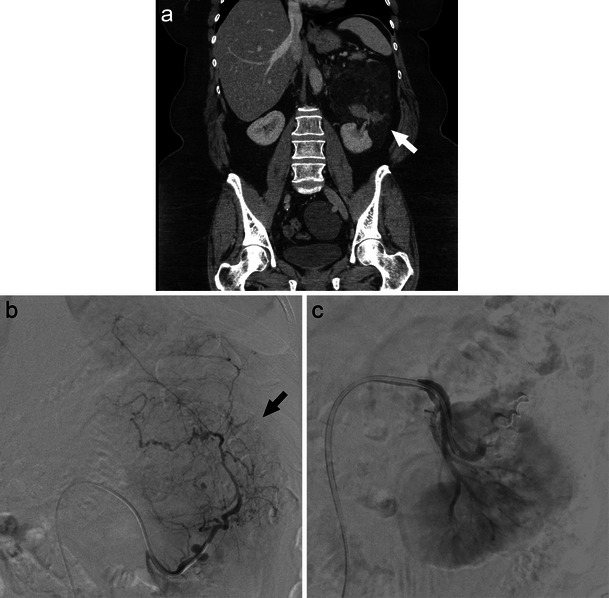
Large left renal AML as seen on CT (a, white arrow) and angiography (b, black arrow) subsequently embolised with sclerosing agent (ethanol) (c)
